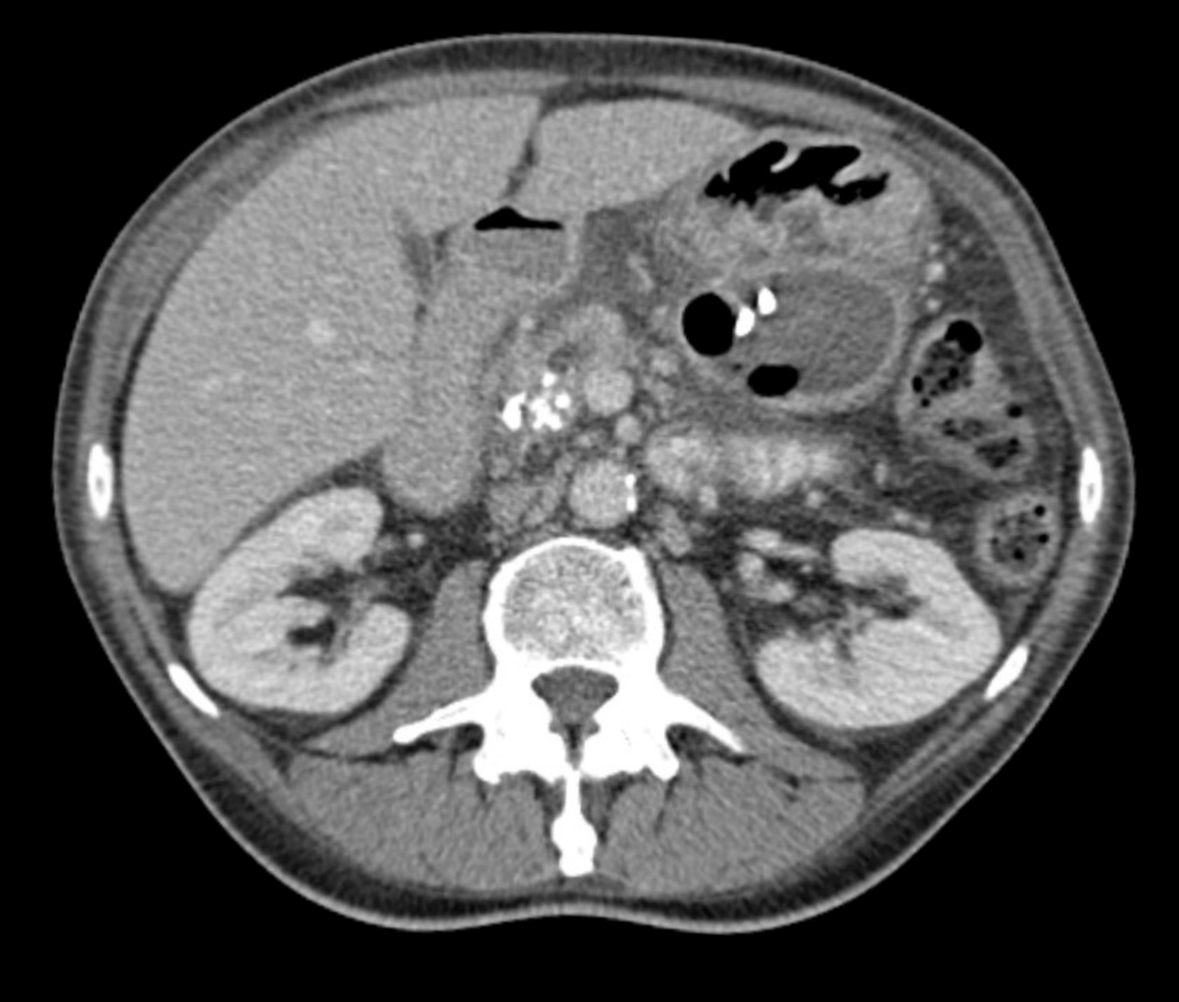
Abdominal CT-scan with venous contrast: Pancreatic pseudocyst with transgastric drainage.
